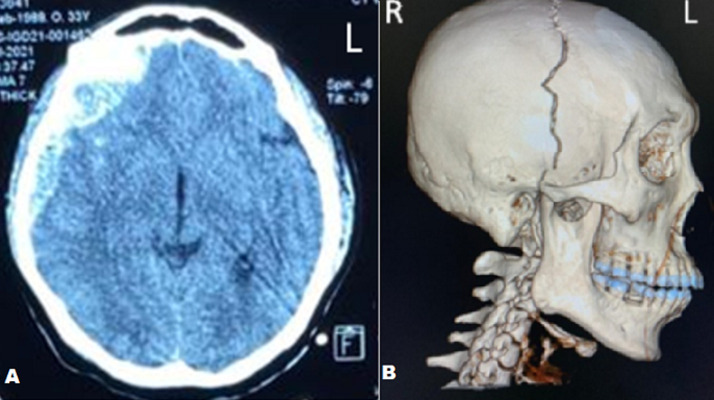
CT scan showing biconvex and crescent in right frontal and temporal region (A); 3D skull radiographic showing coronal suture diastasis widening up to the right temporal bone (B)
